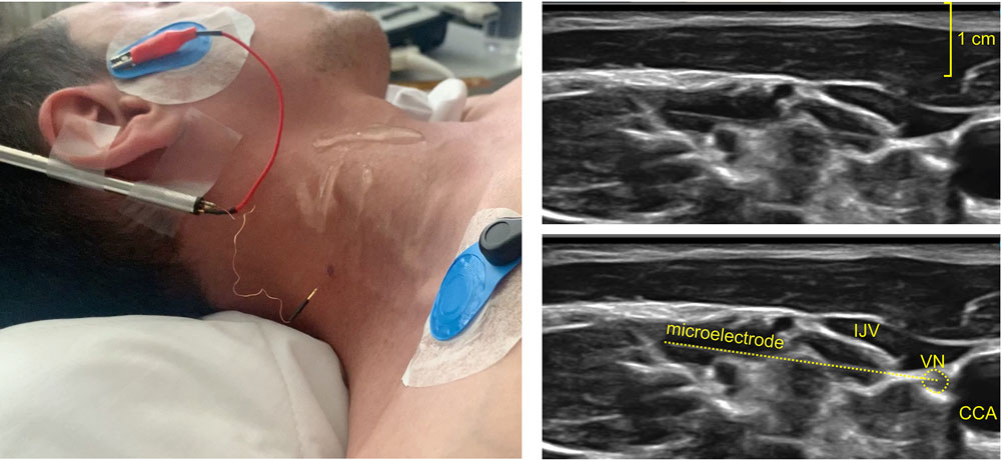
Microelectrode inserted into the right vagus nerve in a 26‐year‐old male breath‐hold diver (BHD 2) performing a maximal inspiratory apnoea (note the distended external jugular vein). Ultrasound gel can be seen on the neck. The ultrasound images at the right show the raw and annotated images of the microelectrode, with the tip in situ within the VN. The nerve is lateral to the CCA and posterior to the IJV. Abbreviations: CCA, common carotid artery; IJV, internal jugular vein; VN, vagus nerve.
